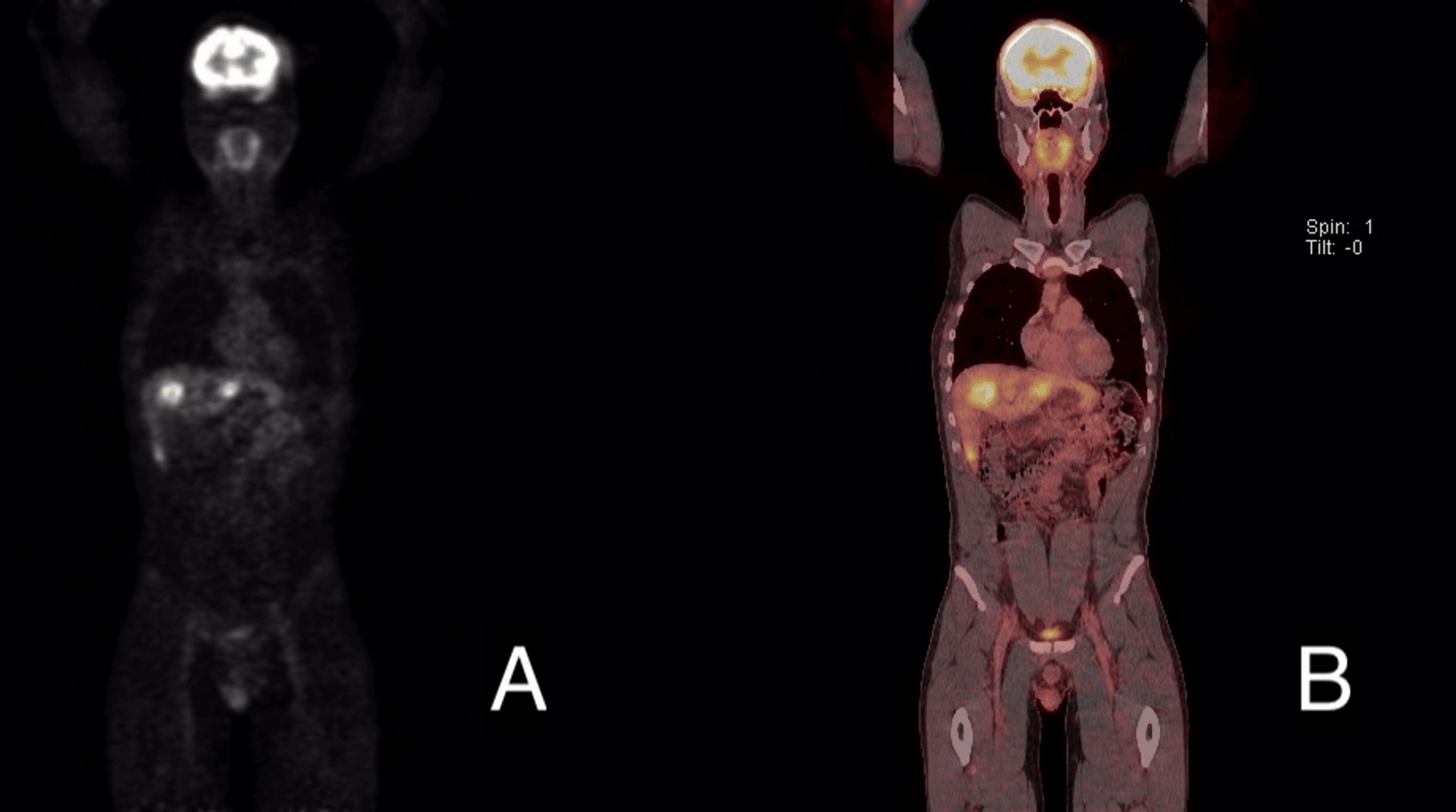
Initial PET-CT coronal plane A: PET image acquisition phase. B: Fusion of PET and CT images. Hypermetabolic bilobar liver metastases are visible in both images, located in segments 8/4a, 2, and 6. PET-CT: positron emission tomography-computed tomography
